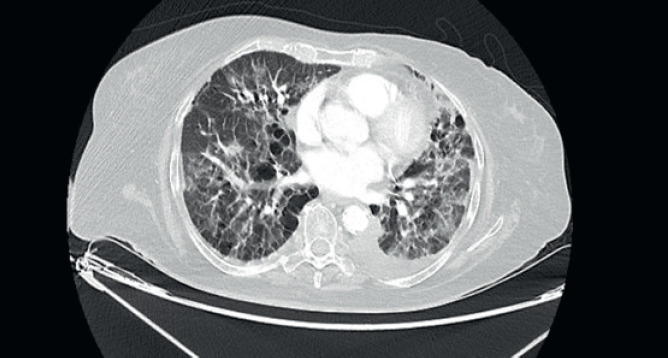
High-resolution CT thorax of the patient, taken at timepoint of clinical deterioration, three months after initiation of CDK 4/6 inhibitor (Palbociclib) therapy.
